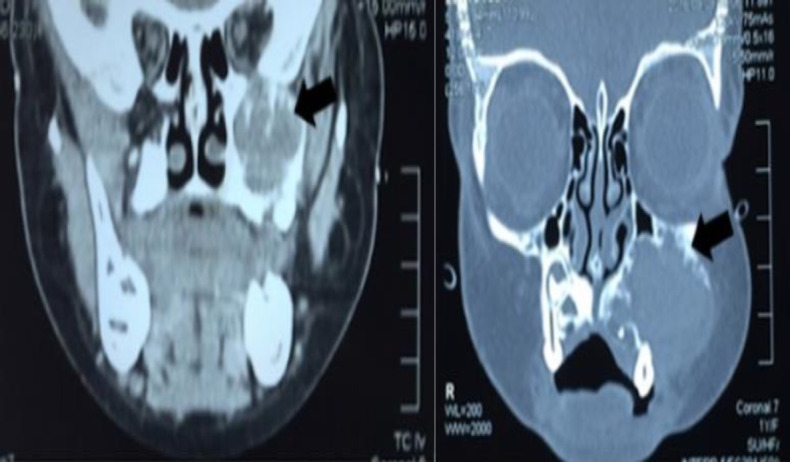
CT of the nose and paranasal sinuses. Shows findings of an expansive lesion in the maxillary sinus cavity that erodes and causes lysis of the maxillary sinus walls
